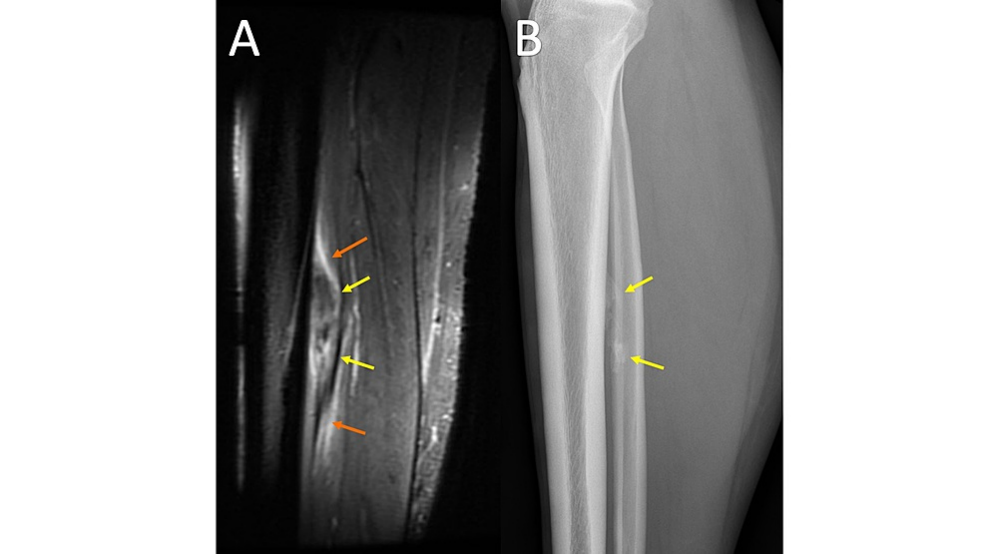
Myositis Ossificans MRI sagittal T2 fat saturation (FS) (8A) obtained at the time of initial presentation demonstrates an ill-defined T2 hypointense region (yellow arrows) with a surrounding T2 hyperintense signal likely representing edema (orange arrows), a non-specific imaging appearance. Lateral tibia/fibula radiograph (8B) shows immature mineralization (yellow arrows), which, especially with the clinical history, aids in the diagnosis of myositis ossificans. A follow-up radiograph six weeks later (not shown) demonstrated the development of peripheral mineralization with central lucency consistent with myositis ossificans.
